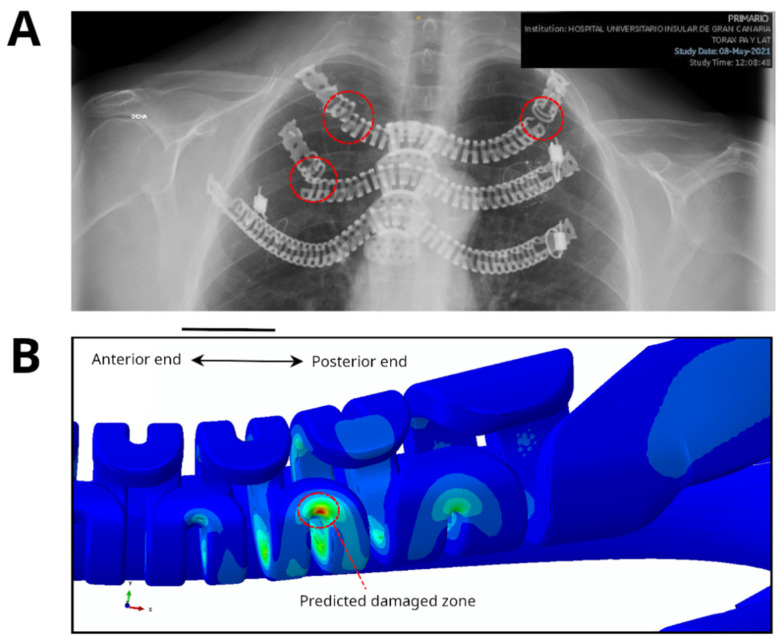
(A) X-ray of a fracture occurring in a clinical case reported in 2018 [20], taken on 8 May 2021, at the Hospital Universitario Insular de Gran Canaria, involving one of the earliest implant designs. (B) Damage region predicted by PF-CZM in the latest improved designs for both the unilateral and bilateral models.
